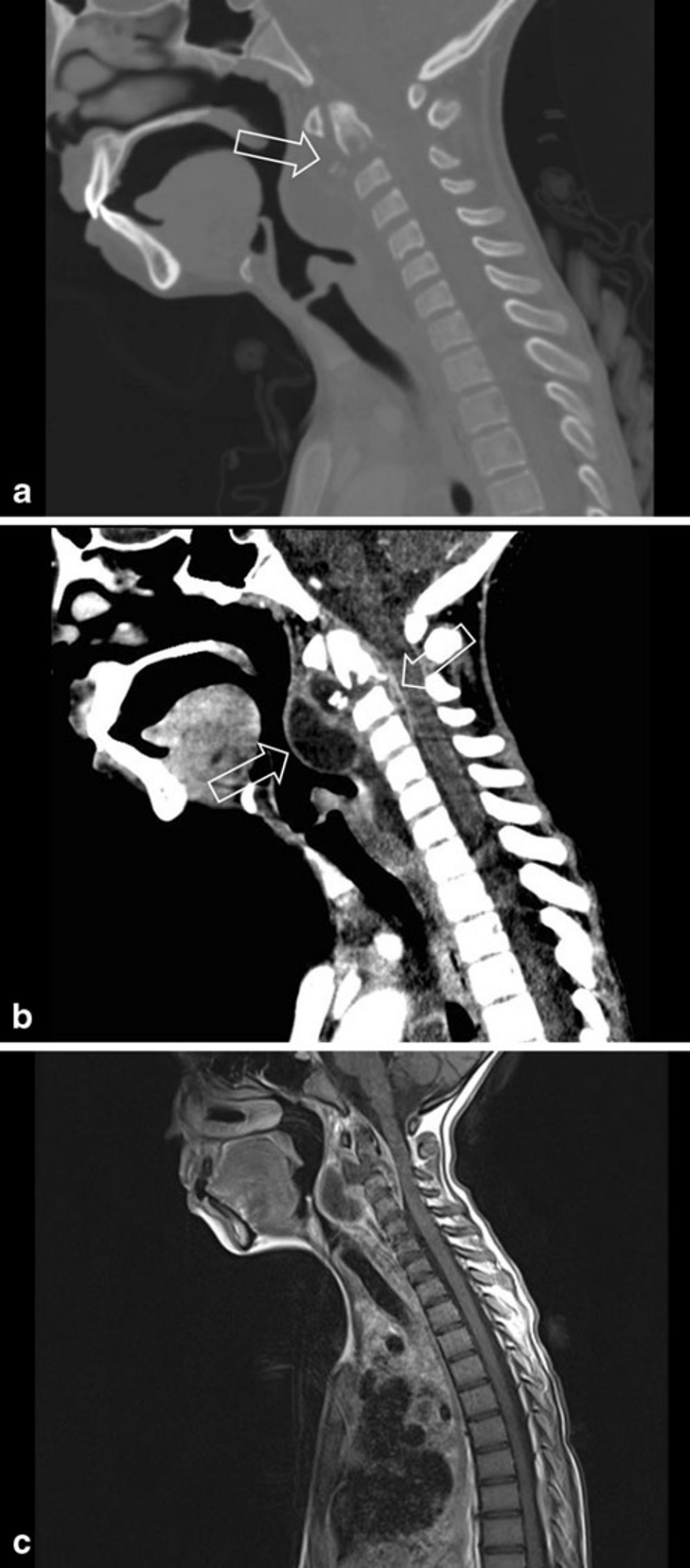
a A 9-year-old girl from Ethiopia, with cervical lymph nodes and weight loss, under suspicion of having tuberculosis. Fluid collection was seen on ultrasound at level II and the patient underwent CT to exclude a retropharyngeal abscess. CT showed multiple cervical and mediastinal lymph nodes, but also destruction of the end plate of the corpus of C2 with a fragment dislocated anteriorly (*arrow*). b CT in the soft tissue kernel clearly shows a prevertebral fluid collection with rim enhancement, but also posterior extension in the spinal canal, with enhancement. The diagnosis is a tuberculous spondylodiscitis. c On the sagittal T1 contrast-enhanced fat-saturated image, one can clearly see the anterior abscess, but also the intraspinal extension of the abscess in the spinal canal
